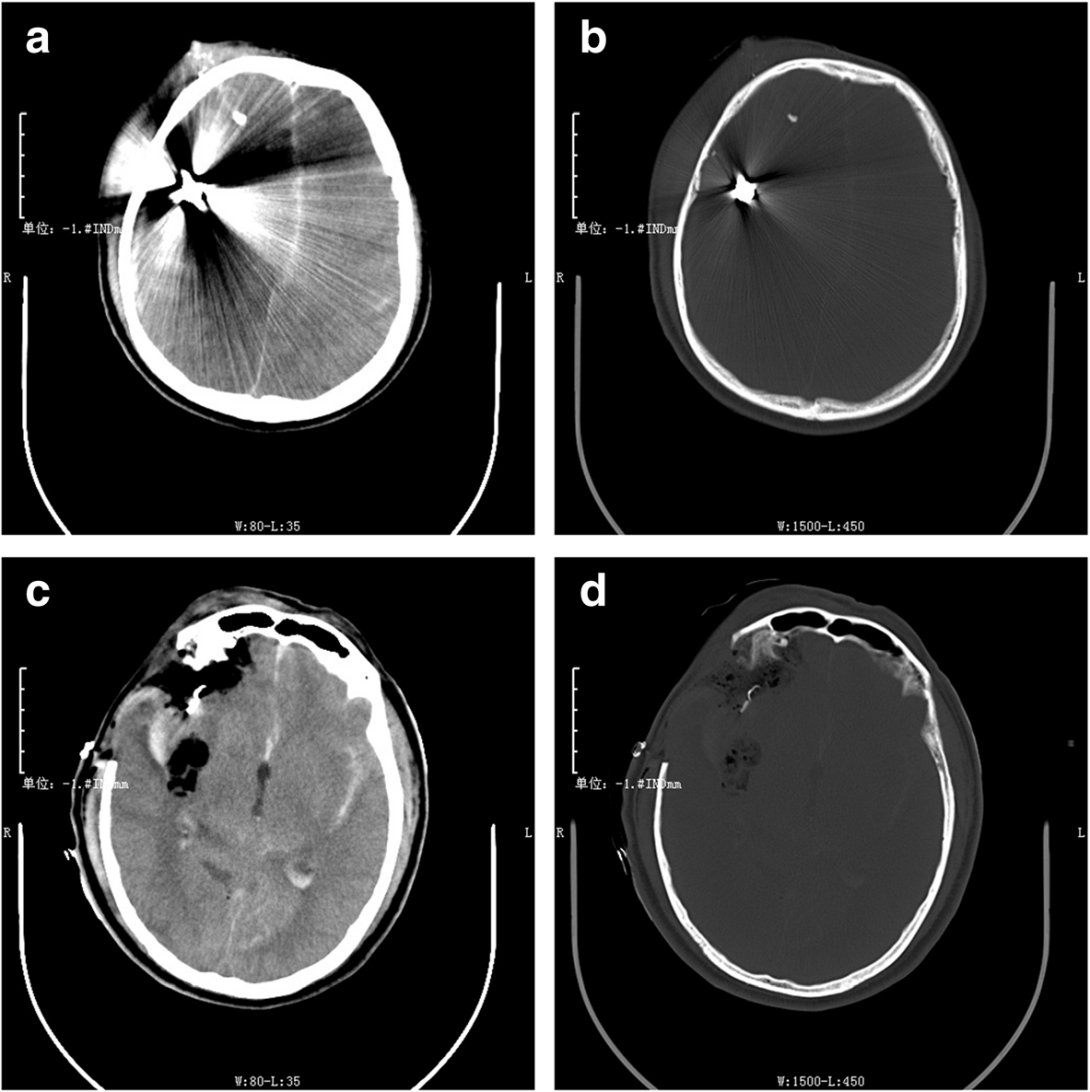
Computed tomography (CT) upon admission. a Soft tissue windows and b bone windows, showing the metal artifacts from the bullet case. Postoperative CT on the same day in soft tissue windows c, and bone windows d after decompressive craniectomy
